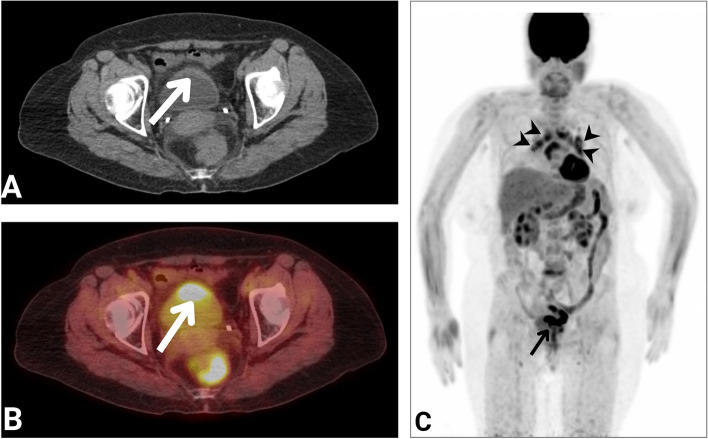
A 69-year-old patient diagnosed with bladder cancer who underwent ^18^F-FDG PET/CT for staging. Axial CT scan (a) revealed anterior bladder wall thickening (white arrow). Axial fused PET/CT images (b) demonstrated intensely hypermetabolic anterior bladder wall thickening (white arrow). The MIP PET scan (c) showed the FDG focus within the urinary bladder (black arrows). Additionally, there was ancillary evidence of bilateral symmetrical hilar lymphadenopathy. This was initially reported as granulomatous disease, which was later validated (black arrow heads)
